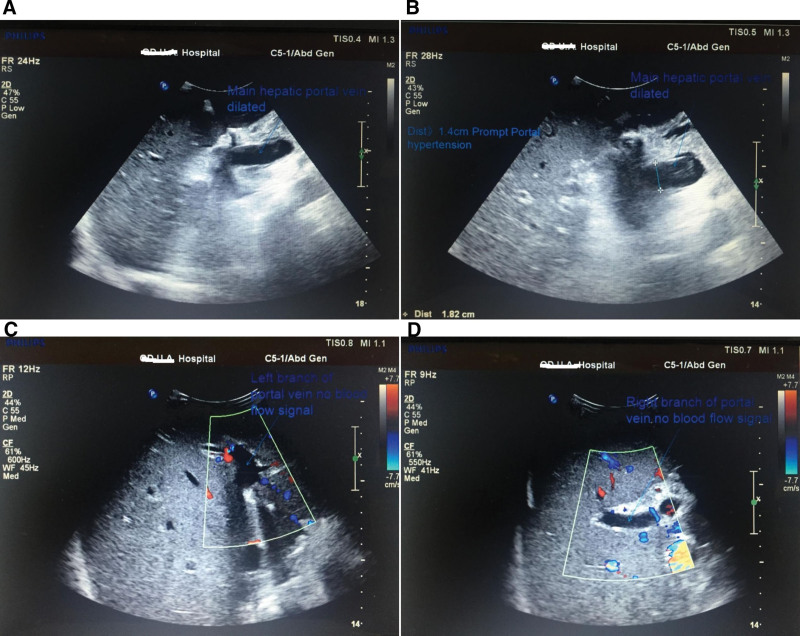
(A) Ultrasound acquisition map of hepatic portal vein dilation. (B) Ultrasound acquisition map of hepatic portal vein dilation (dist > 1.4 cm). (C) Ultrasound acquisition map of hepatic portal vein dilation (left branch of portal vein no blood flow signal). (D) Ultrasound acquisition map of hepatic portal vein dilation. (Intraoperative ultrasonography shows that the new liver was large and hard with an abnormal echo and solid change. Portal vein expansion, high tension, great resistance, and almost no flowthrough; the hepatic artery flow rate is <20 cm/s, and the hepatic vein has no blood flow.)
